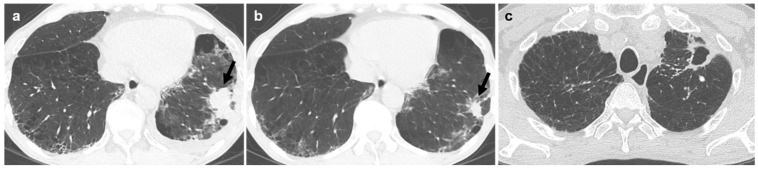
A male patient with nontuberculous mycobacterial infection. (a) Chest CT demonstrates a 3.6 cm lobulated irregular nodule (arrow) in the left lower lobe. Poorly differentiated carcinoma was diagnosed via percutaneous core needle biopsy. (b) On CT scan obtained 6 months earlier, the lesion measured 1.7 cm (arrow). Emphysema was present in underlying lung. (c) A fibrocavitary pattern of nontuberculous mycobacterial pulmonary disease is present in the left upper lobe.
